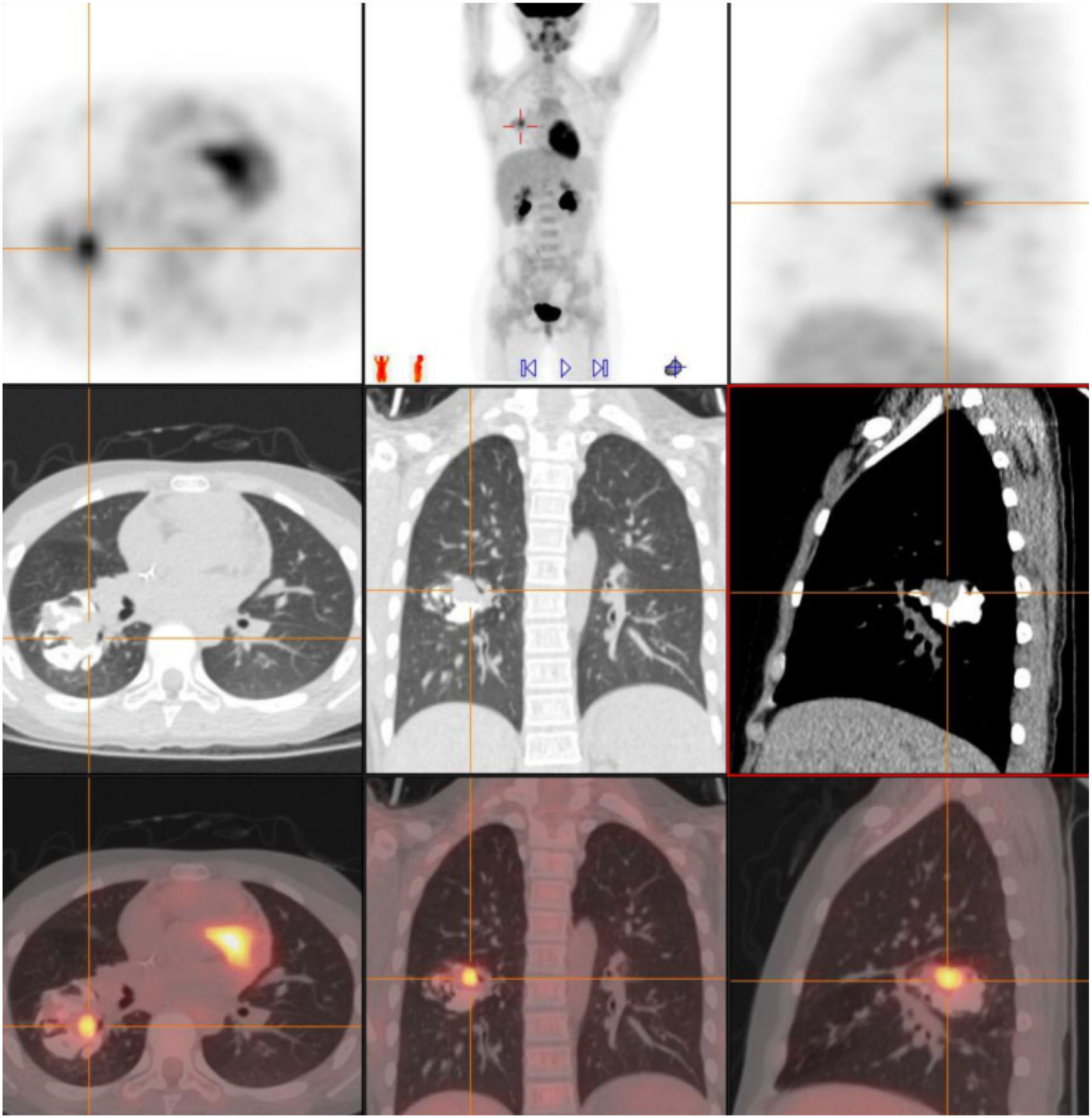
Preoperative PET-CT findings. Preoperative PET-CT demonstrating a heterogeneous mass in the dorsal segment of the right lower lobe (33 × 32 × 27 mm) with coarse calcifications and uneven FDG uptake (SUVmax: 4.9). The lesion caused partial bronchial narrowing, but no abnormal FDG uptake was detected in the mediastinal lymph nodes or distant organs, suggesting localized disease.
